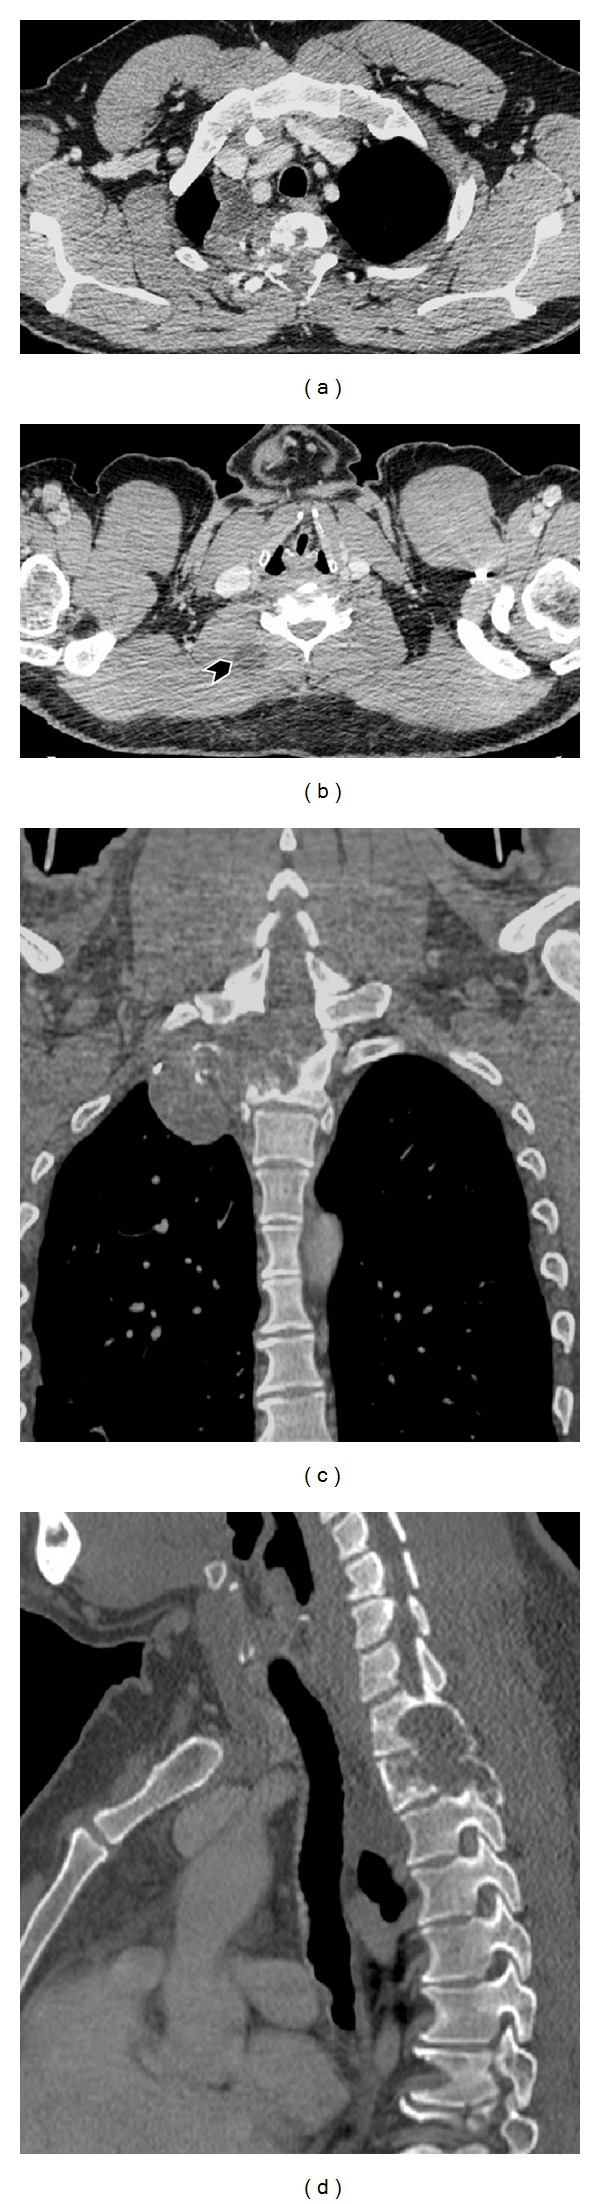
CT exam after intravenous contrast injection: axial (a) and (b), coronal (c), and sagittal (d) planes demonstrate cystic tissue localized in the chest and in the paravertebral muscles on the right (arrow head) and bone erosion of second rib and D1 and D2 vertebrae.
